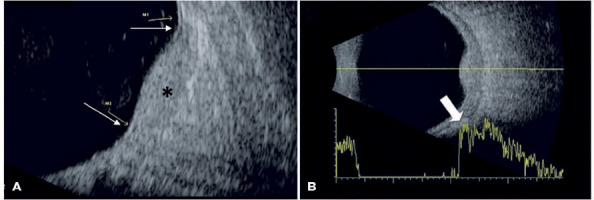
10 MHz ultrasound of a circumscribed choroidal hemangioma (*). (A) A
